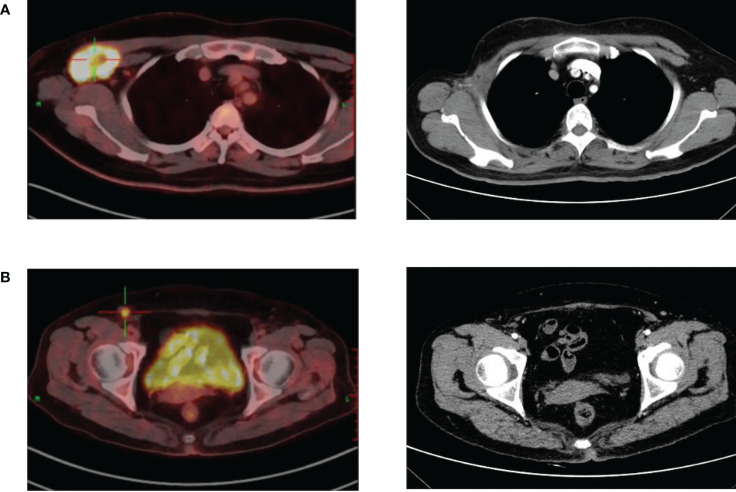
(A) An enhanced CT of the chest showed no recurrent manifestation in the right axilla compared with preoperative PET-CT. (B) CT follow-up revealed the disappearance of the lesion in the right inguinal region after adjuvant radiotherapy compared with preoperative PET-CT.
